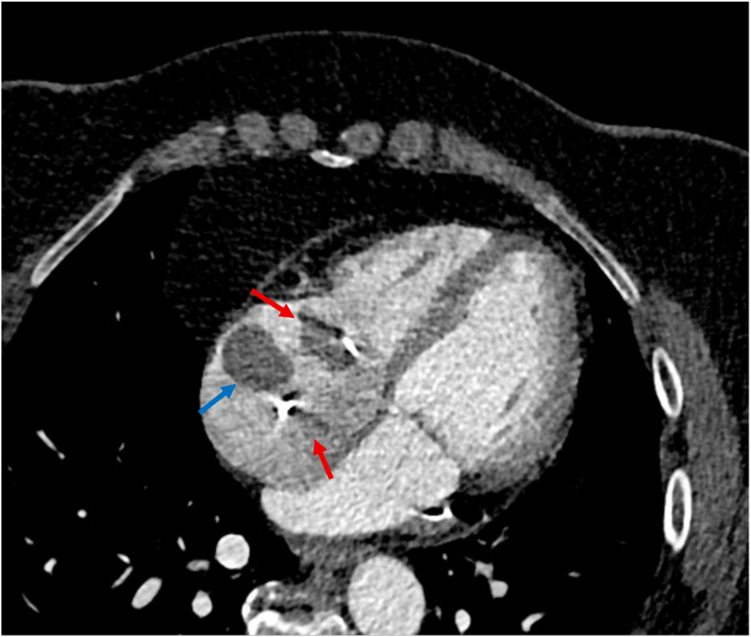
Contrast-enhanced computed tomography scan of the aorta showing 3 hypodense filling defects (arrows) within a dilated right atrium, the largest (blue arrow) appears adherent to right atrial free wall and maximum diameter measured up to 2.9 cm.
